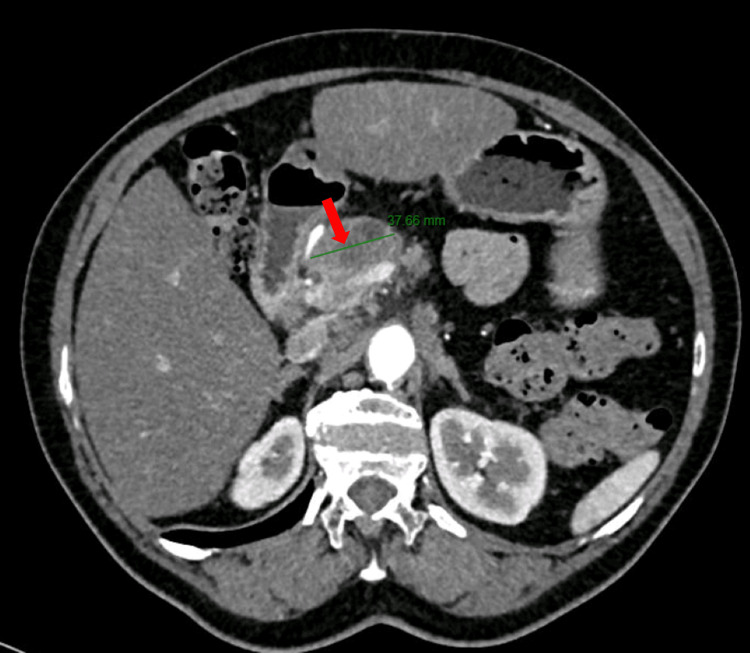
Abdominal computed tomography showing a 3.7 cm (greater axis) infiltrative lesion of the head of the pancreas (red arrow)
